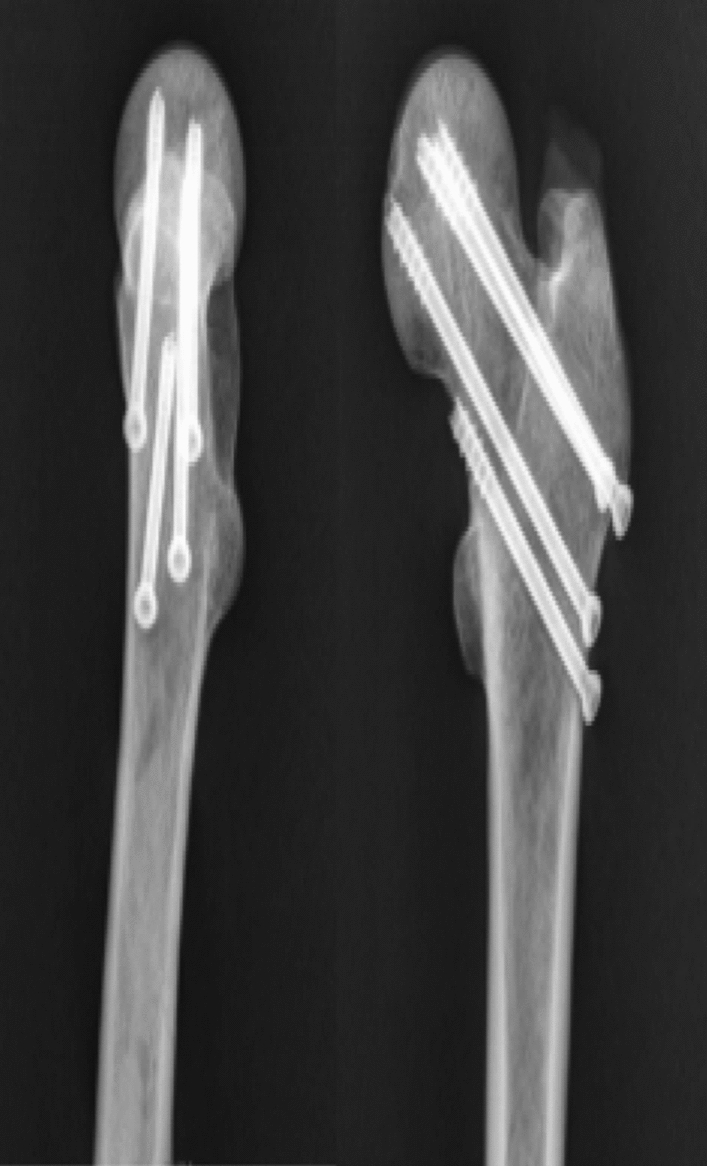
Medial femoral neck support screw combined with three cannulated compression screws (3CCS + mFNSS) (*Note*: Positive and lateral X-ray film after internal fixation).
